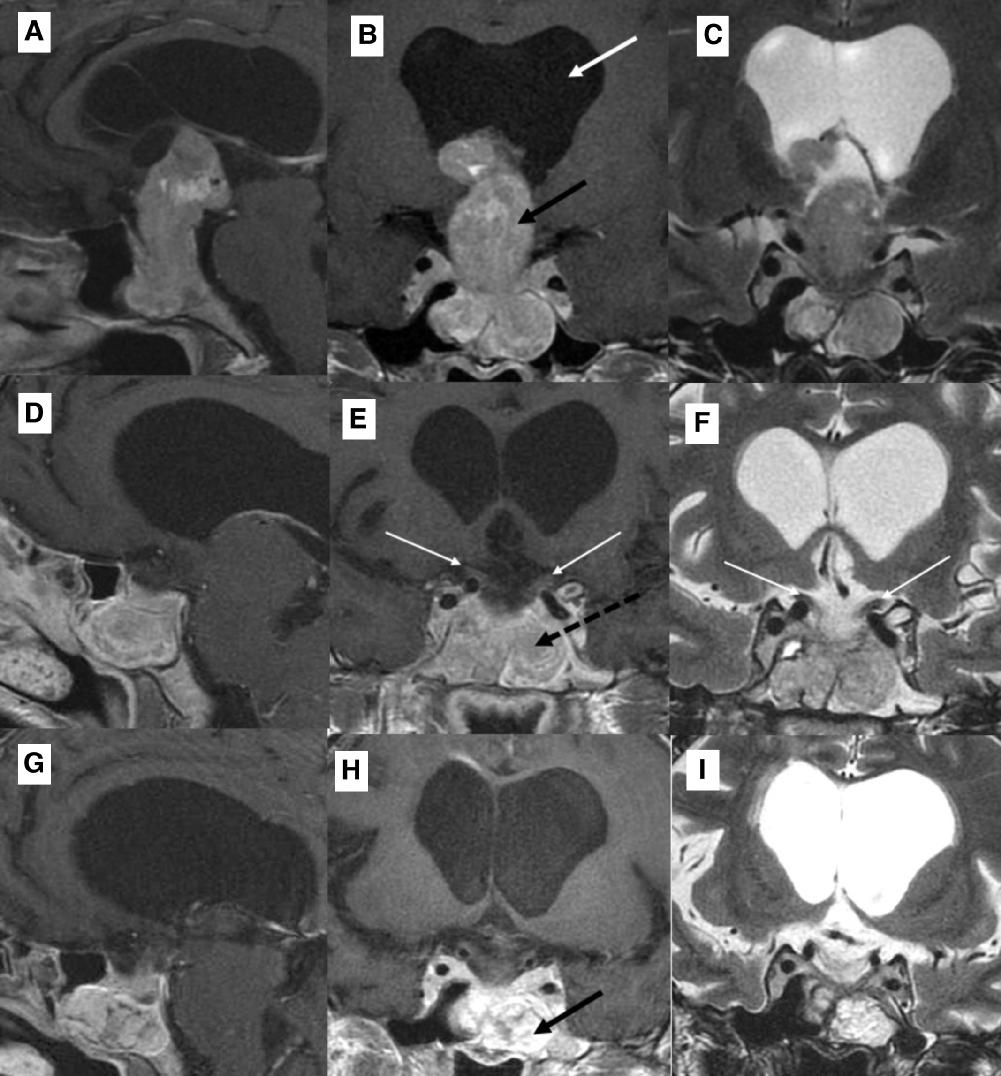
Sellar MRI. Sagittal T1 post-contrast (A, D, G), coronal T1 post-contrast (B, E, H), coronal T2 (C, F, I) views. (A-C) Preoperative MRI showing a 5.1 × 3.1 × 2.2 cm sellar, supra-, infra-, and para-sellar mass (black arrow) that occupied the sphenoid sinuses inferiorly and invaded both cavernous sinus. The lesion had significant vertical growth, invaded the third ventricle, and caused obstructive hydrocephalus (white arrow). The lesion displayed heterogeneous contrast enhancement, was slightly hyperintense on T1 and T2, and was suggestive of a pituitary macroadenoma. The optic chiasm, pituitary gland, and stalk were not individualized. (D-F) Three months after the first surgery, residual mass could be seen in the sphenoid sinus (dashed black line) with the optic chiasm decompressed and pulled down (white arrows). (G-I) One month after the second surgery, residual lesions in both cavernous sinuses could be seen, as well as fat graft that was inserted during surgery for hemostasis (black arrow). Abbreviations: MRI, magnetic resonance imaging.
